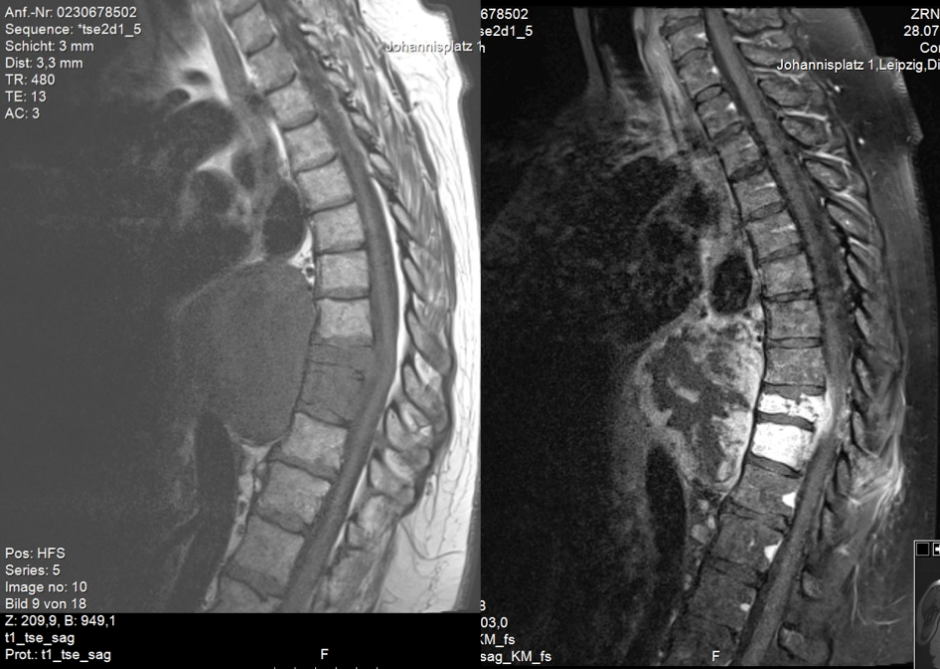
Sagittal MR image of the thoracic spine. Left: T1-weighted image. The tumor represents as a hypointense mass infiltrating the spine with spinal canal penetration. Right: T1-weighted, contrast enhanced image. Inhomogeneous gadolinium distribution.
